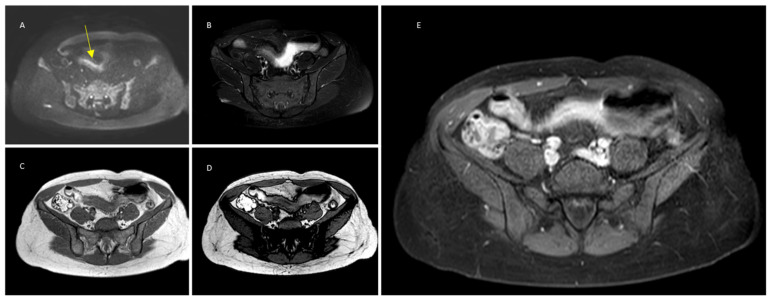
(A) DWI on high b-value image showing the bright luminal sign at the ileal tract (yellow arrow). (B) T2w fat-saturation image shows no signs of submucosal edema. (C,D) T1 IP-OOP showing diffuse submucosal adipose infiltrate. (E) Post-contrast image with homogeneous pattern of enhancement at 7 min.
